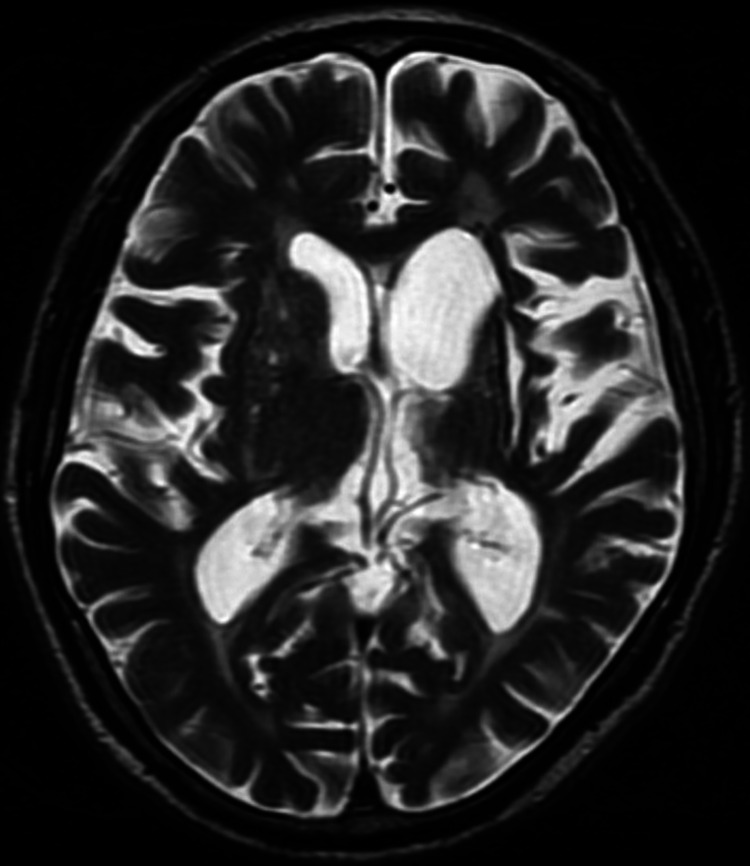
Magnetic resonance imaging (MRI) brain - a slice of the T2 sequence showing stable gliosis in the left basal ganglia with ex-vacuo dilatation as well as old infarcts in the right basal ganglia
